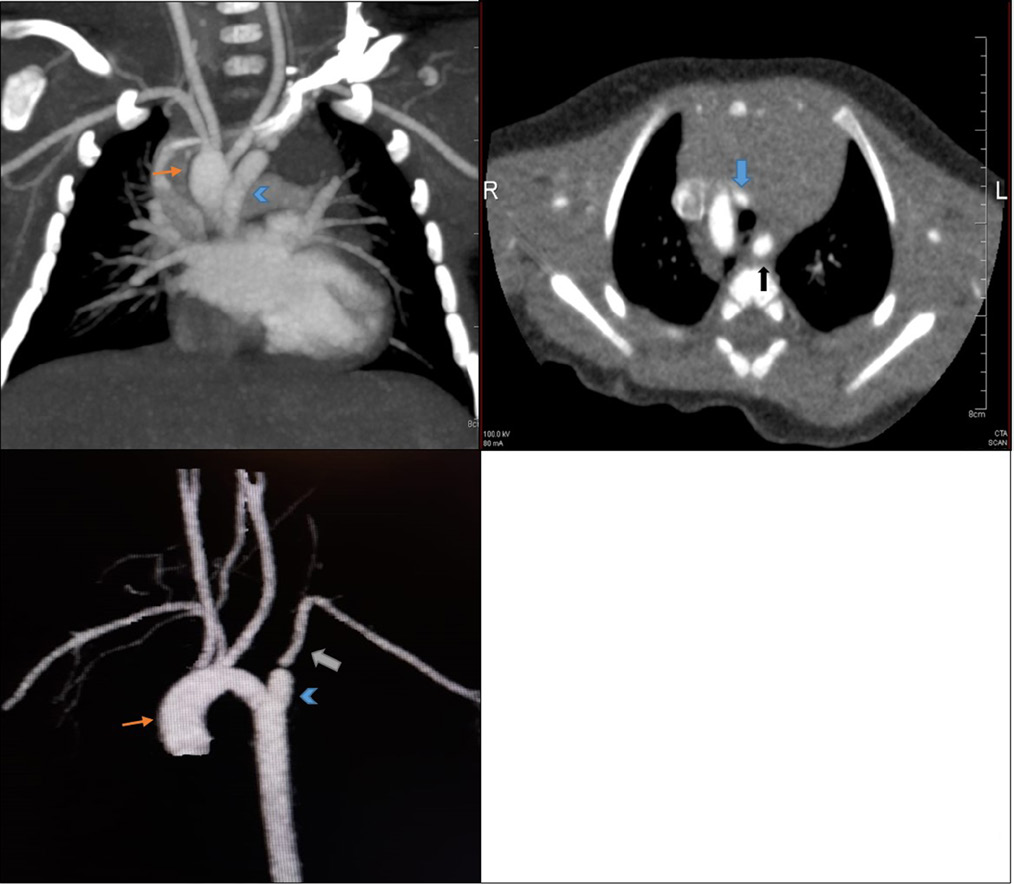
CT angiography (a) coronal, (b) axial and (c) 3D reconstructed views show right-sided aortic arch (orange arrow) with aberrant left subclavian artery ALSA (white arrow) which arises from a dilatation at its origin (KD; arrowhead). Left CCA arising from right-sided aortic arch coursing anterior to the trachea (blue arrow), ALSA courses posterior to oesophagus (black arrow) on the left side-making a vascular ring.
